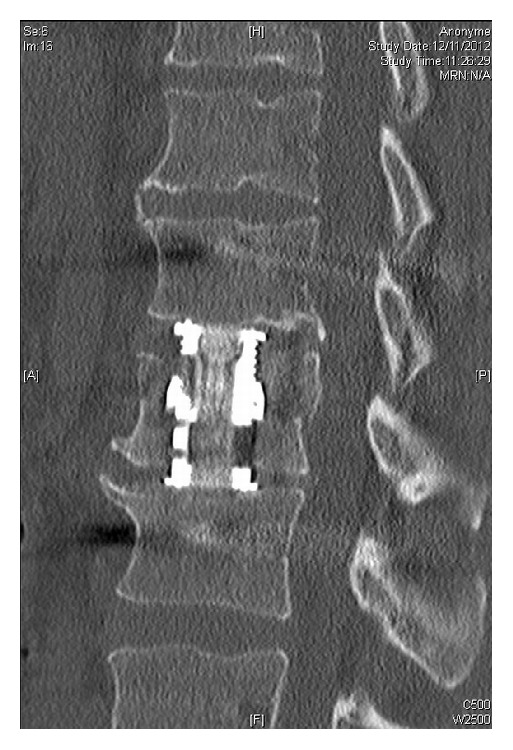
Sagittal postoperative CT-scan showing results after posterior percutaneous osteosynthesis and anterior body graft using an expandable body prosthesis.
